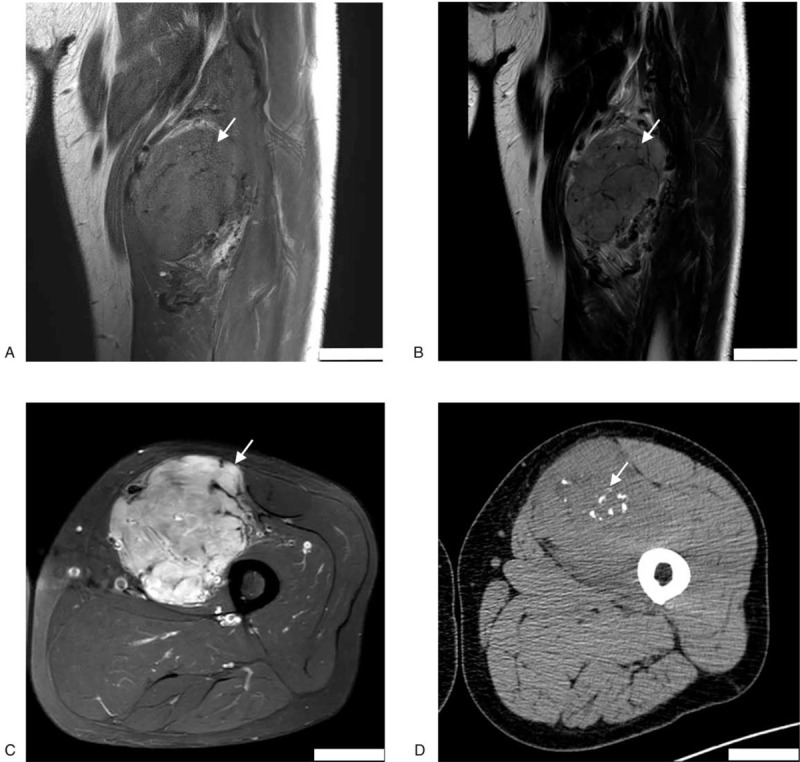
MRI and CT imaging of histiocyte-rich rhabdomyoblastic tumor. The T1-weighted (A), T2-weighted (B), MRI and CT (C) demonstrated a tumor in the left thigh. Several calcifications were observed in the tumor (D). CT = computed tomography, MRI = magnetic resonance imaging.
